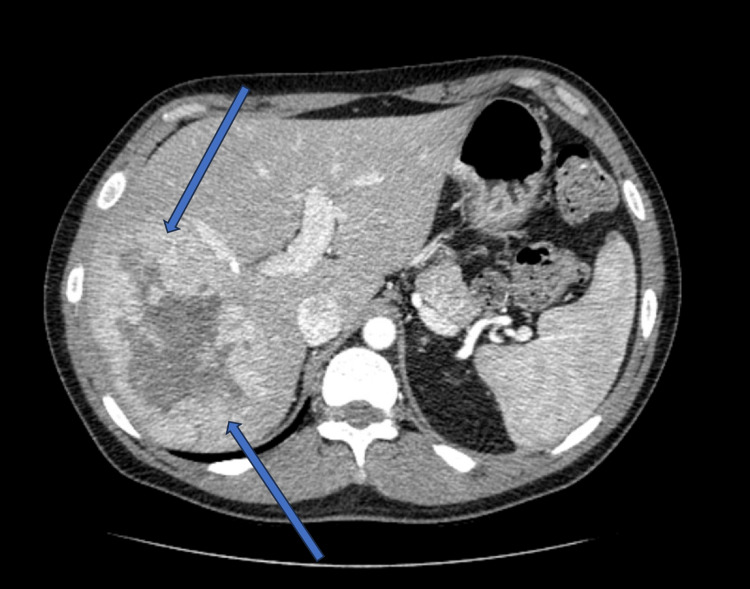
CT scan of the abdomen and pelvis with IV contrast A large hepatic lesion in the right lobe, measuring approximately 10.0 x 6.4 x 10.4 cm, with heterogeneous attenuation.
